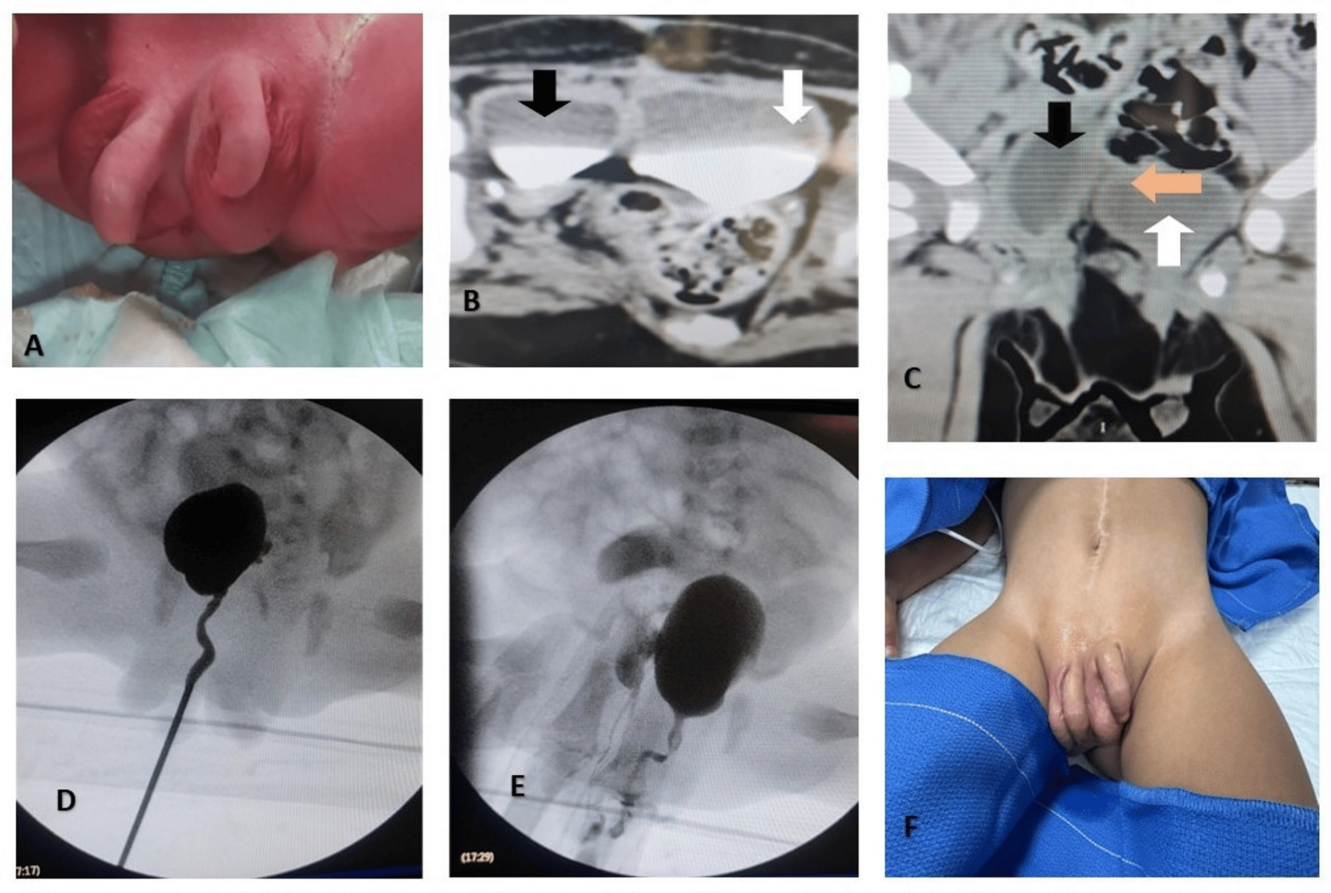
Clinical presentation (A) Genitourinary examination showing diphallia with two fully formed penises, bifid scrotum containing one testis in each hemiscrotal compartment, and duplication of the anal orifice. (B) Axial CT urography in the excretory phase showing duplicated urinary bladders, each receiving drainage from a single ipsilateral simple renal collecting system; right bladder (black arrow) left bladder (white arrow). (C) Coronal CT scan demonstrating bladder duplication with a well-defined septum (orange arrow)  separating the two vesical cavities; right bladder (black arrow) left bladder (white arrow). (D) Right-sided cystogram. (E) Left-sided cystogram. (F) Postoperative recovery after resection of an accessory right colon segment.
